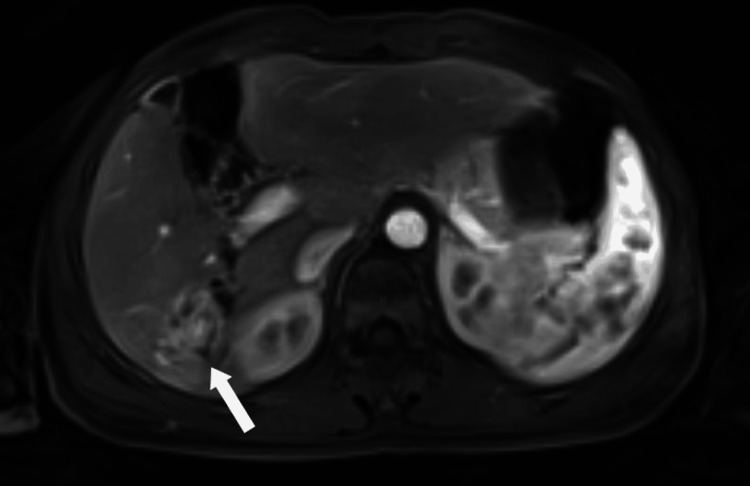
Axial contrast-enhanced MRI in the early arterial phase at the level of the lesion Contrast-enhanced MRI in the early arterial phase showing early enhancement of the intraductal lesion (white arrow).
